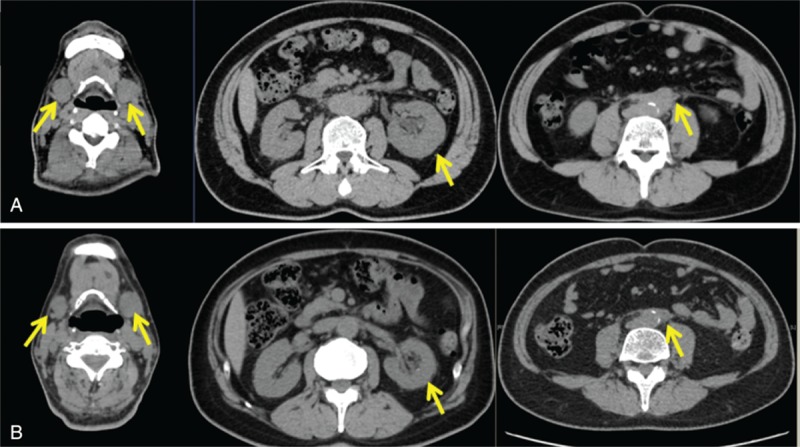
Computed tomography (CT) findings before and after glucocorticoids therapy: (A) CT findings before glucocorticoid therapy and (B) CT findings after glucocorticoid therapy. The swelling of submandibular glands (left), periaortitis (right), and left kidney (middle) was improved after glucocorticoids therapy (yellow arrows).
